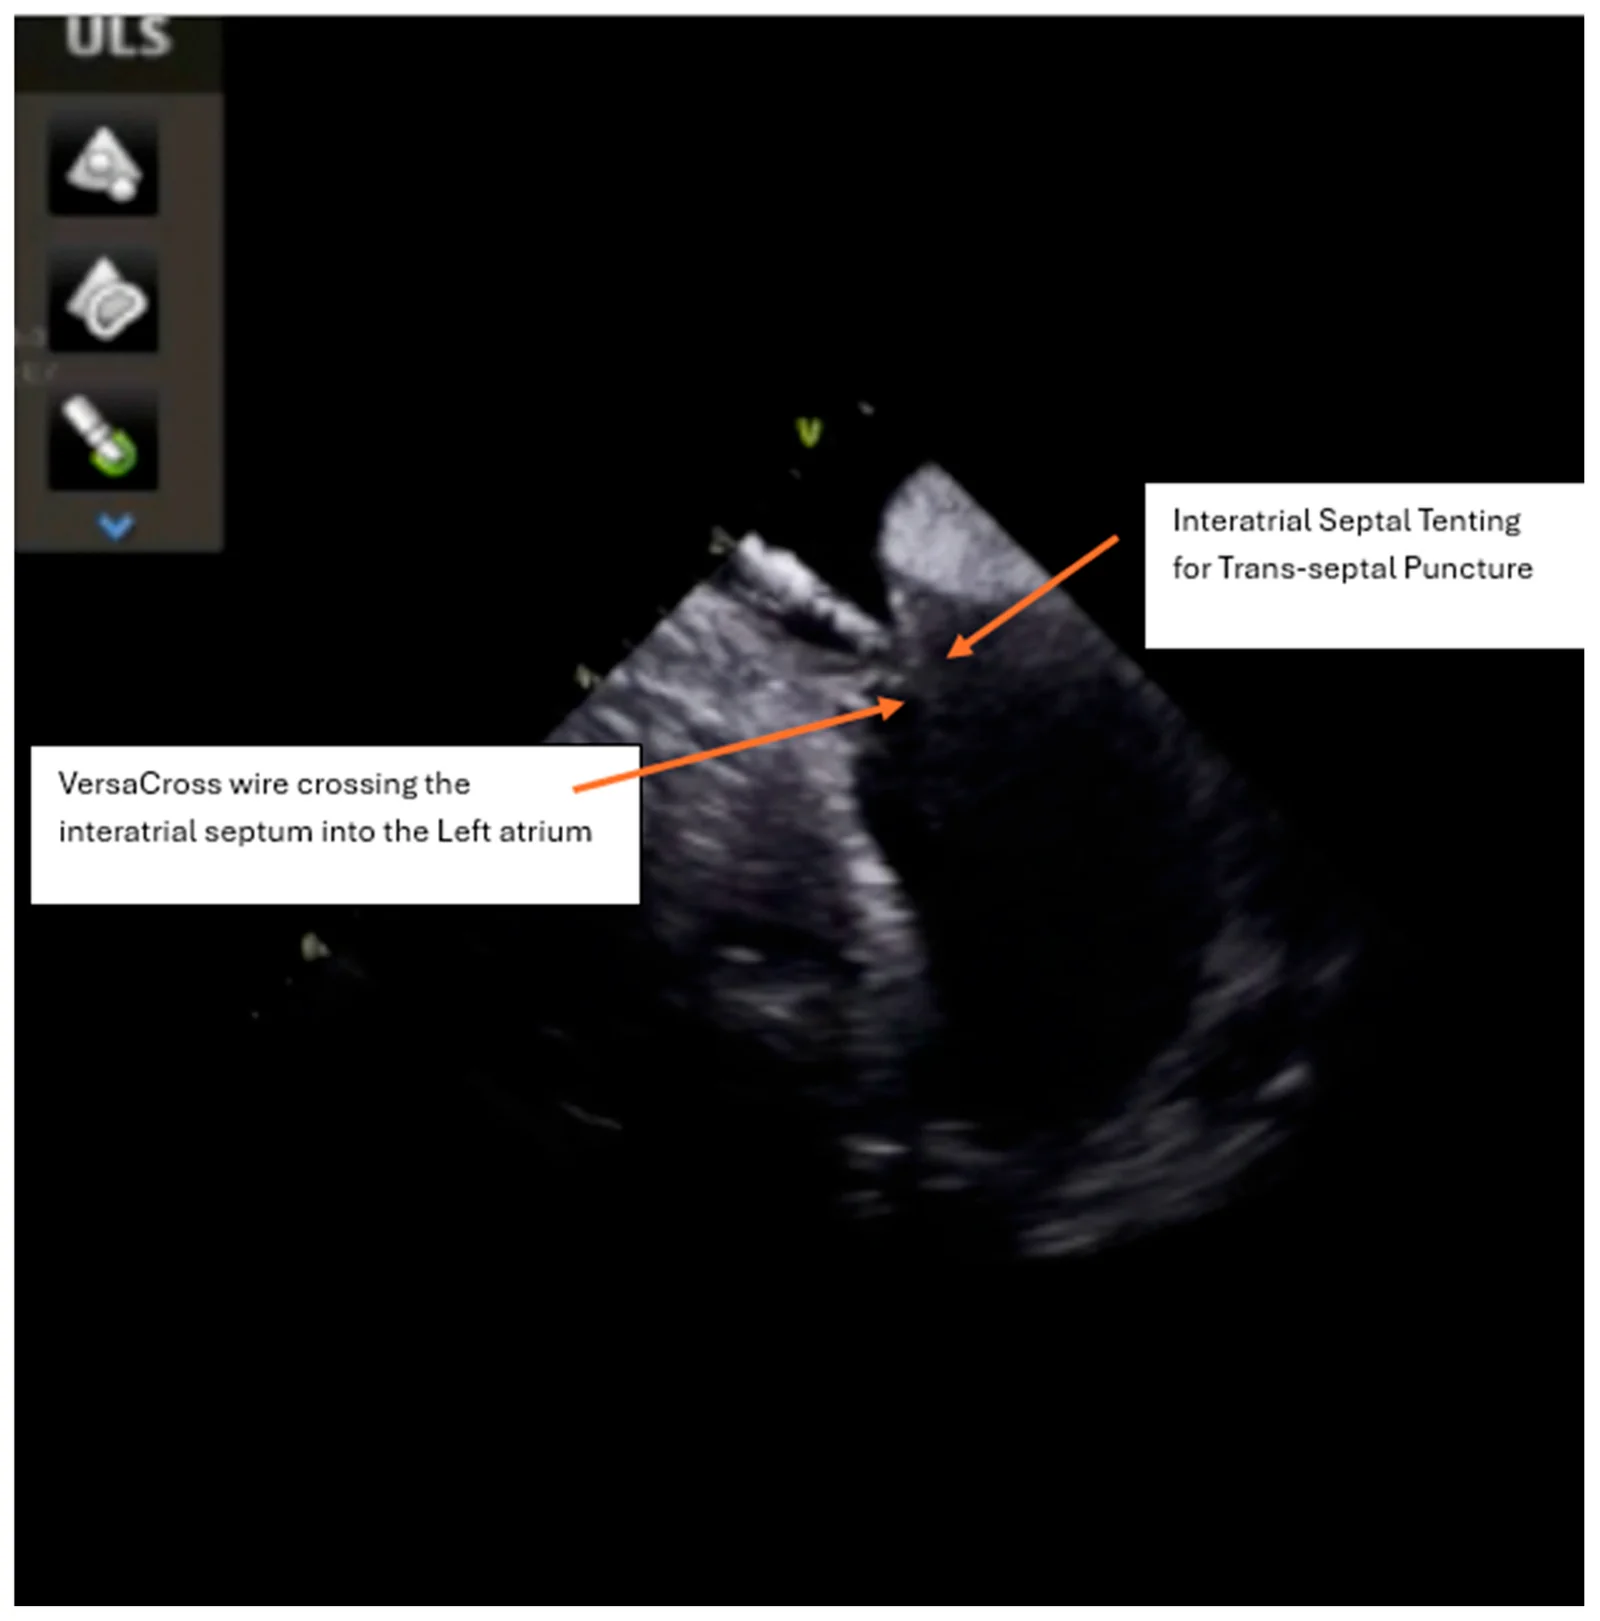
Fluoroless transseptal puncture under intracardiac echocardiography (ICE) and CARTO 3 guidance. Septal tenting at the mid-posterior, slightly inferior portion of the interatrial septum (arrow) with the radiofrequency-enabled VersaCross wire advanced across the interatrial septum into the left atrium (arrowhead).
